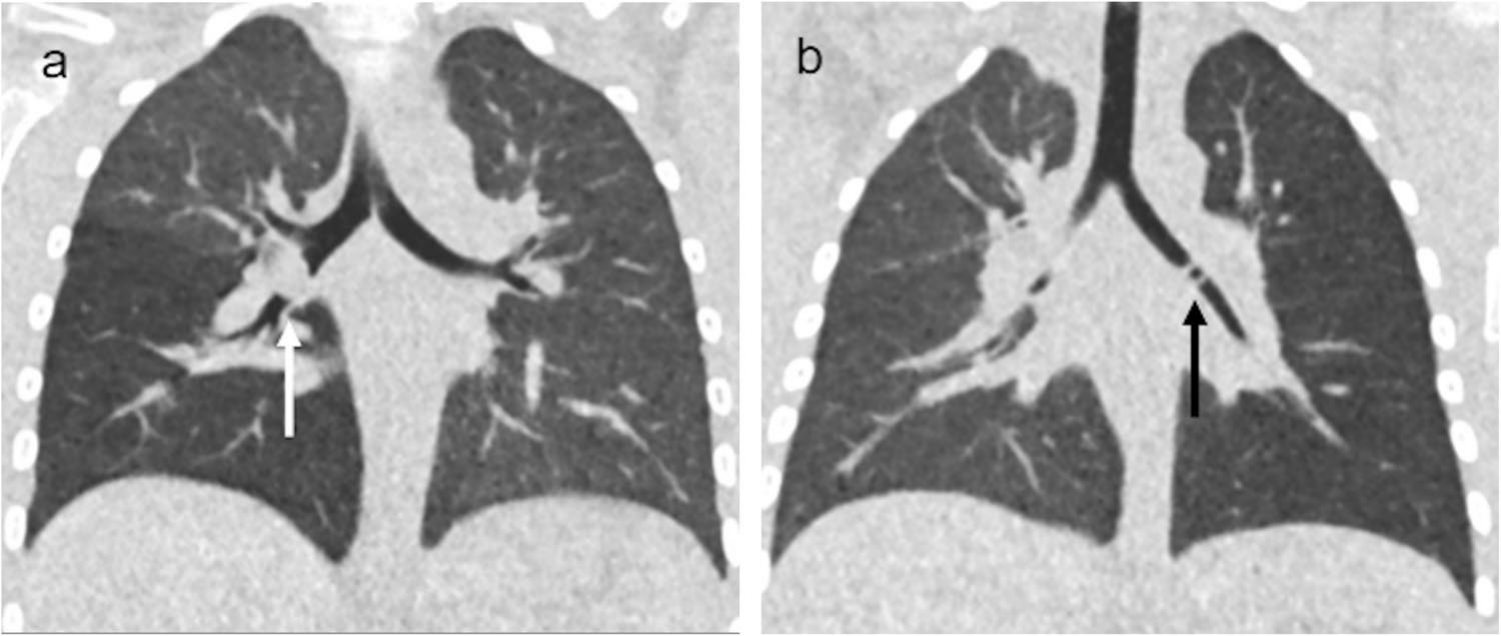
a Coronal view computed tomography (CT) without contrast, in lung window, of a 7-year-old girl with a piece of carrot in the right bronchus (*white arrow*). b Displays a coronal view CT without contrast, in lung window, of a 2-year-old with a Perler bead in the left main bronchus (*black arrow*). Both examinations had an effective dose of 0.04 mSv. Republished from *Diagnosis of a foreign body aspiration with ultralow-dose CT using a tin filter: a comparison study,* Courtesy of Dr Lena Gordon Murkes [14]
